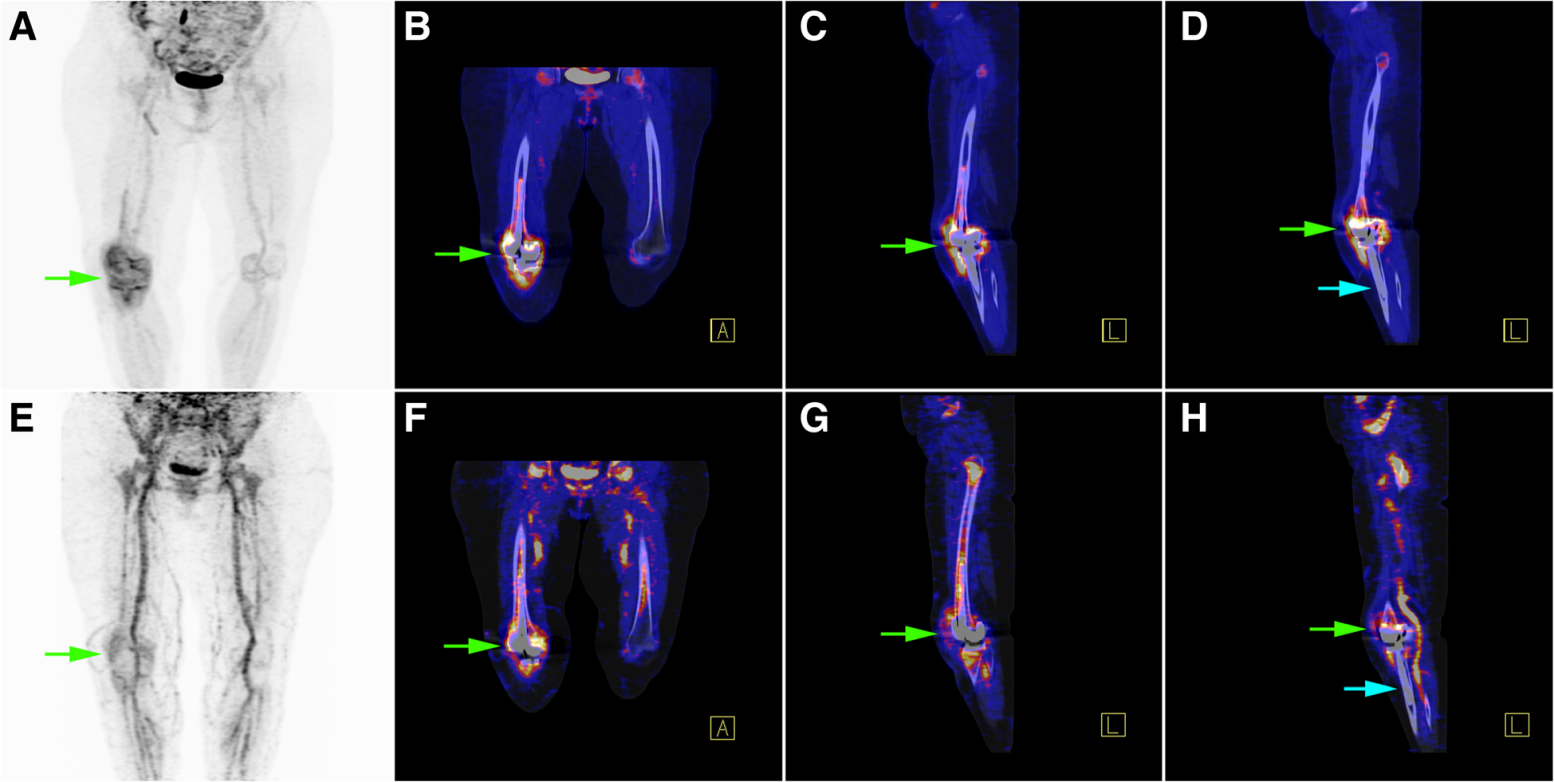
Patient (case # 3) with a history of knee prosthesis implantation performed 12 months before imaging. The results of ^18^F-FDG PET/CT (upper row) and ^68^Ga-citrate PET/CT (lower row) are presented. a–d The green arrows indicate an increased ^18^F-FDG uptake at both the bone prosthesis interface and the knee synovium. e–h A similar pattern of ^68^Ga-citrate uptake was evident. The imaging results were considered positive, and the case was subsequently confirmed as true positive. d, h In contrast to the femoral portion, there was no increased radiotracer uptake along the bone prosthesis interface of the elongated tibial stem component (indicating no loosening or infection of a specific prosthesis segment, blue arrows)
